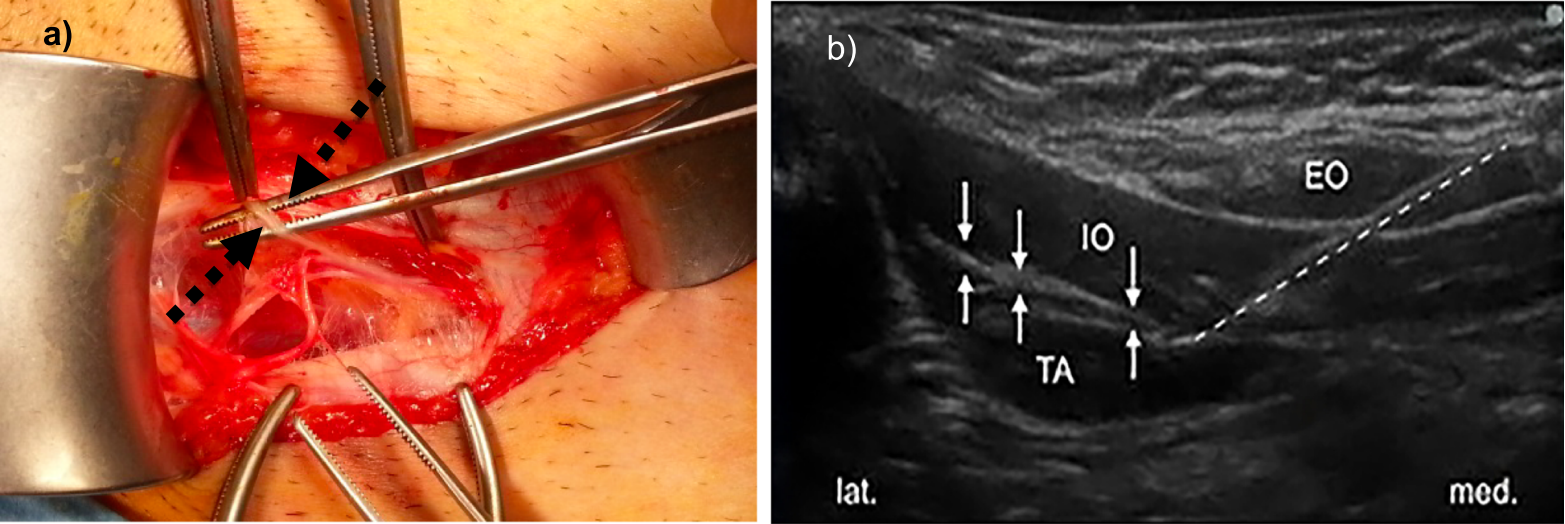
Anatomy: a macroscopic: Black arrows = ilioinguinal-iliohypogastric nerves; b Ultrasound image: EO = abdominal external oblique muscle, IO = abdominal internal oblique muscle, TA = transverse abdominal muscle; White arrows = Ilioinguinal and iliohypogastric nerves between fasciae, Dotted line = needle in situ; lat.=lateral, med.=medial
